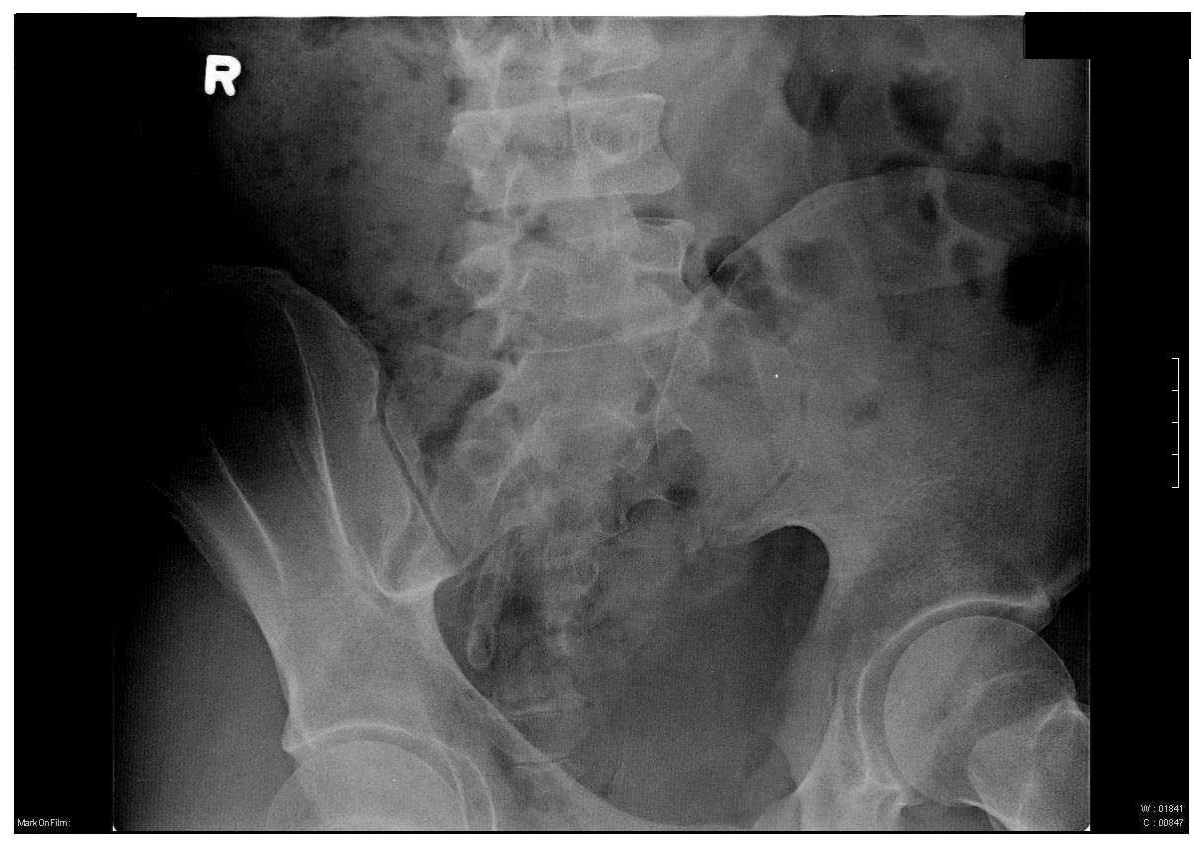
Sacroiliac joints x-ray showing narrowing and irregularity of the left sacroiliac joint.
